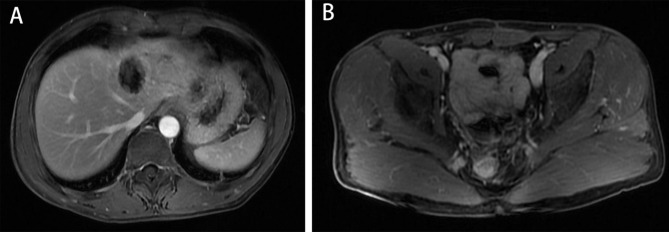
The latest imaging data A-B: MRI showed no metastatic disease or local progression
